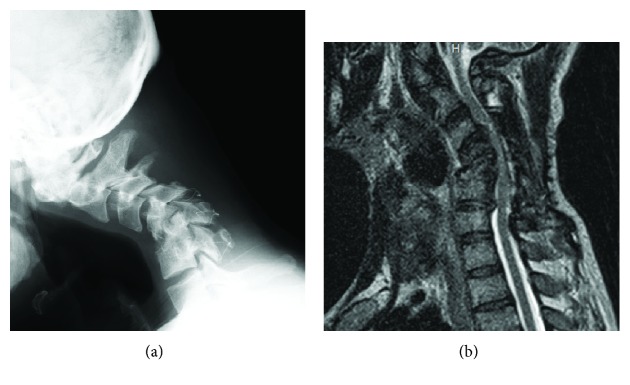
(a) X-ray of the cervical spine obtained 1 year after the initial surgery revealed progression of the destructive changes, with a C2-7 lordotic angle of -53°. (b) Sagittal T2-weighted MRI of the cervical spine performed one year after the initial surgery showed that the spinal cord was compressed by multisegmental stenosis with kyphotic deformity.
